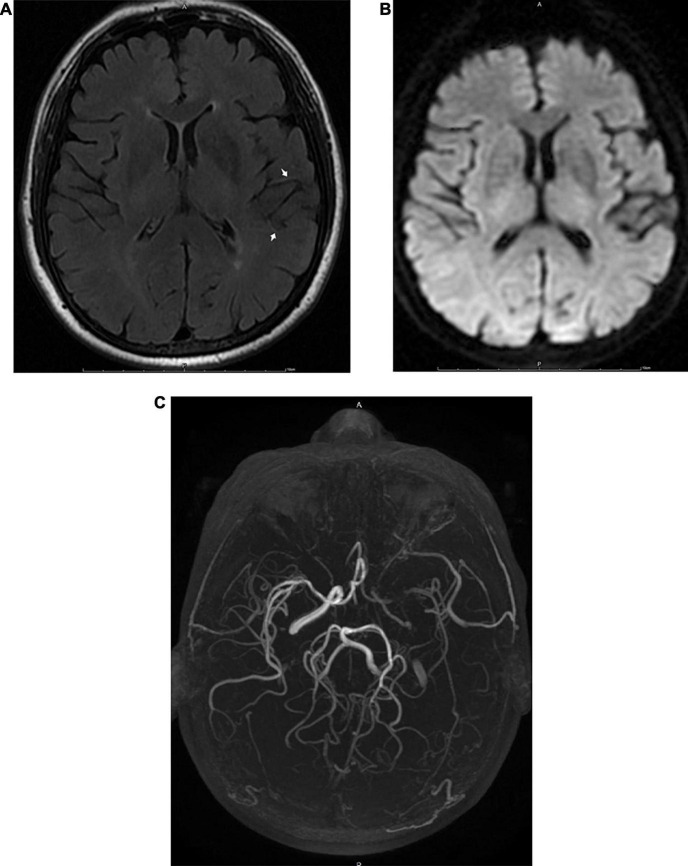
MRI images obtained 6 h after the appearance of the initial symptoms in a 68-year-old male patient with transient ischemic attack. A FLAIR image (A) shows FVH in the territory of the left MCA (arrow). There were no abnormalities on DWI (B). Three-dimensional time-of-flight MRA (C) shows occlusion of the ipsilateral MCA and ICA.
